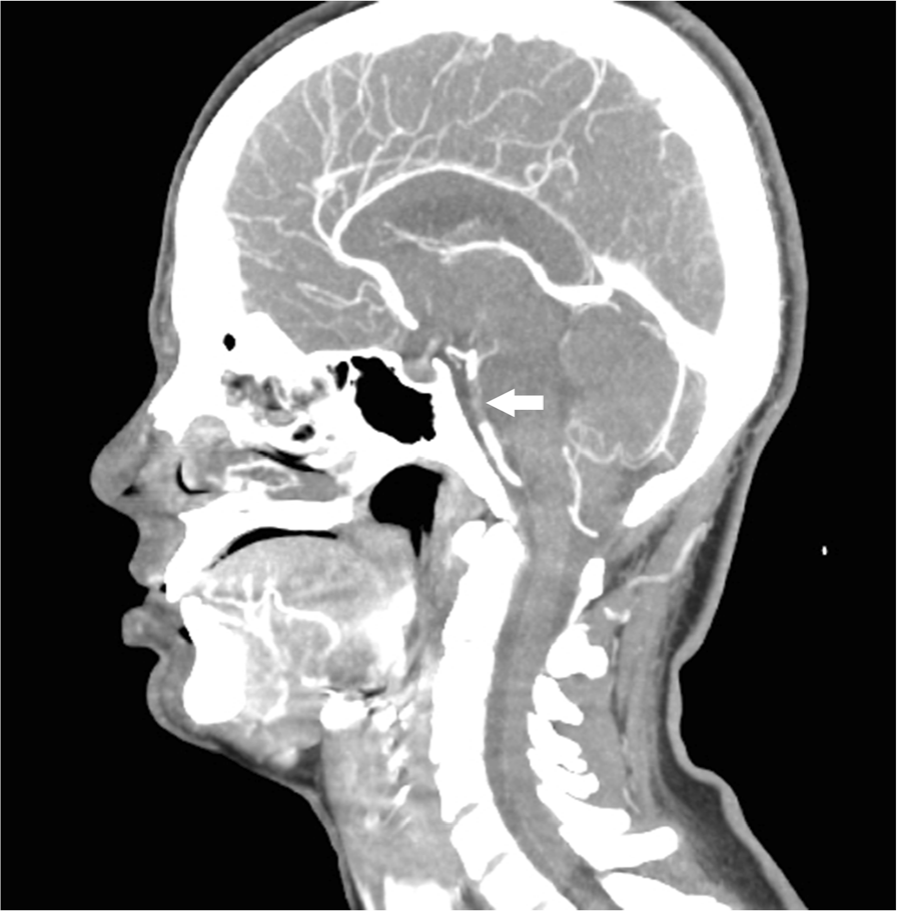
CT angiography (*sagittal view*) revealed a 1-cm filling defect of the basilar artery (*arrow*)
